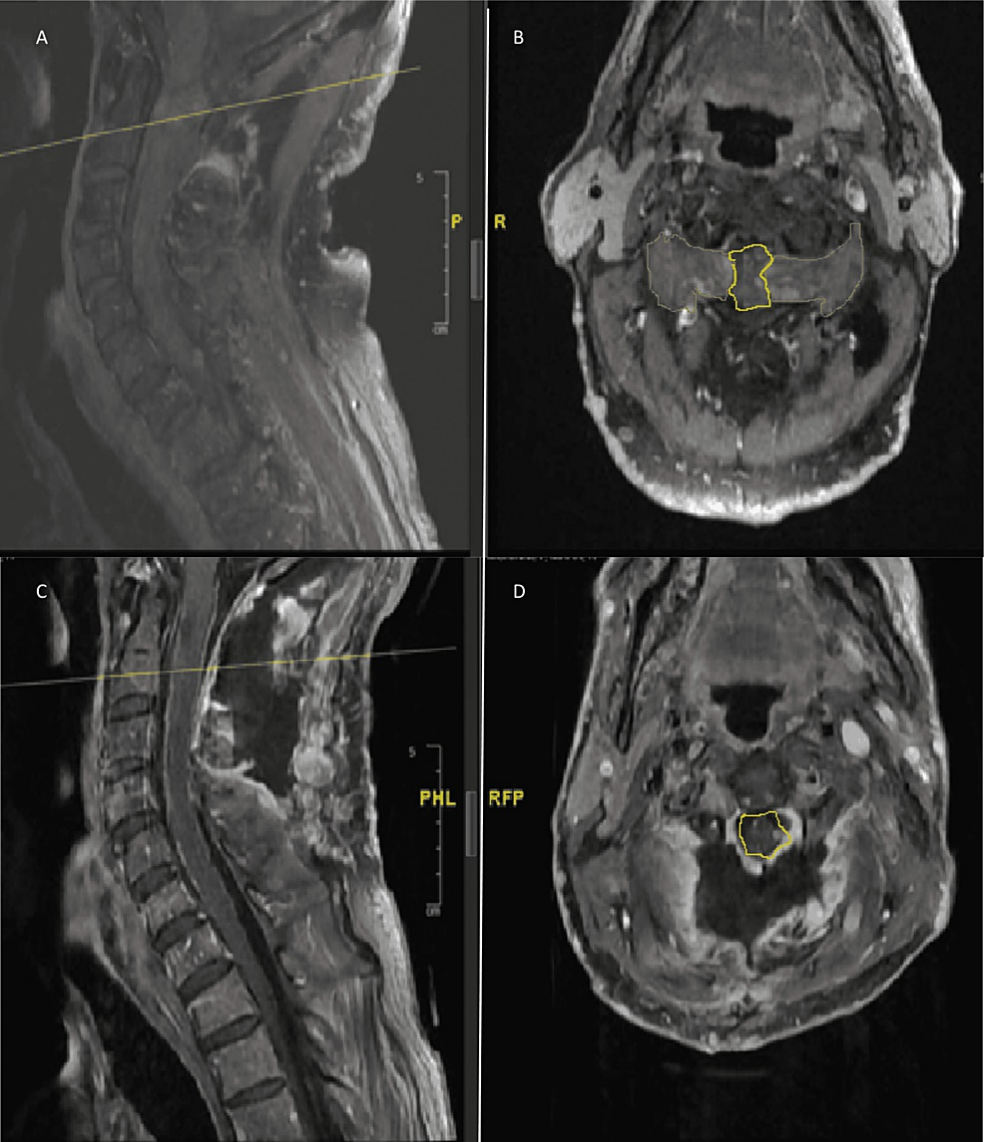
MRI T1 fast Sat post-contrast images of mid-sagittal (A) and axial (B) of the bilateral neurofibromas pre-operatively (C) and post-resection (D). Note the margins of bilateral tumors are indicated by yellow lines and the resultant severe compression of the spinal cord (yellow margins). The margins of the spinal cord in 1D demonstrate the relation of the spinal cord after decompression.
